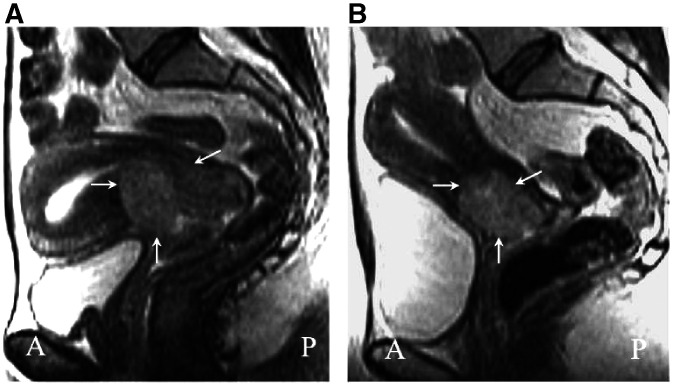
Sagittal T2-weighted fast spin-echo (FSE 3000/88 ms (TR/effective TE)) images before (A) and after (B) three cycles of neoadjuvant chemotherapy. The intermediate signal intensity tumour confined to the cervix (arrows) reduced from 50 to 18 cm^3^ in volume.
